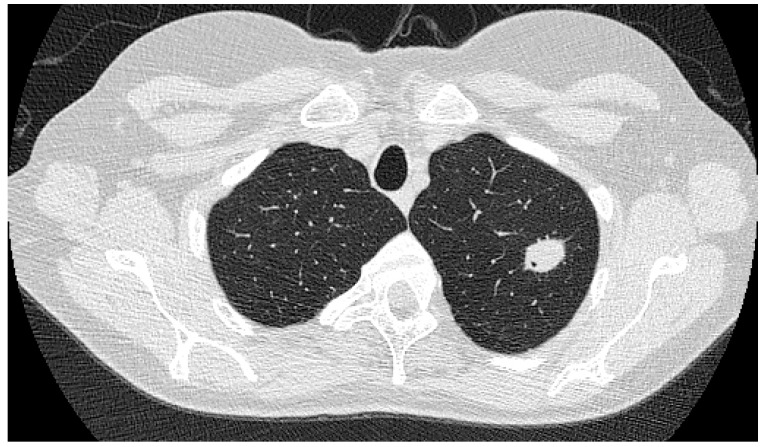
Aspergillus* nodule—CT scan from a patient with an isolated pulmonary nodule attributable to *Aspergillus* spp., unusually this lesion is showing signs of early cavitation.
